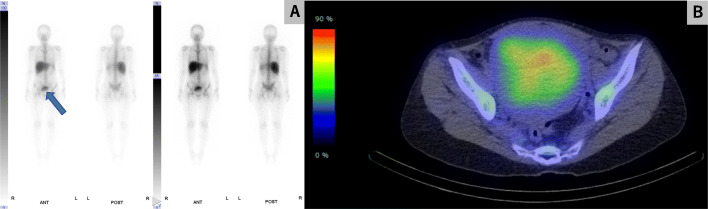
Gallium-67 (^67^Ga) scintigraphy of the uterus after prednisone treatment. ^67^Ga scintigraphy revealed high uptake in the intrauterine cavity (arrow), which suggested an active infection in the uterus. High ^67^Ga uptake was not observed in other organs. a anterior and posterior images of ^67^Ga scintigraphy; b horizontal view
